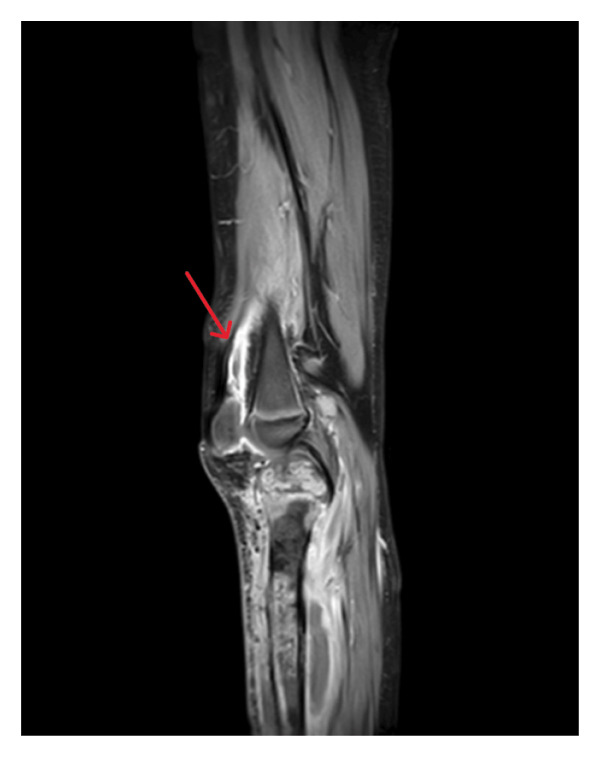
MRI of the left knee (T1‐weighted postcontrast) demonstrating a suprapatellar fluid collection with a thick, enhancing wall, consistent with an abscess.
